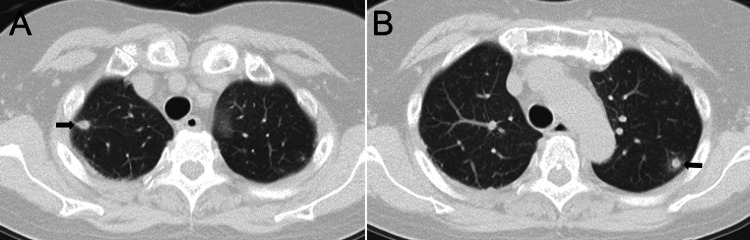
Chest CT A, B: Multiple nodules are observed in the peripheral lungs, with surrounding ground-glass opacities suggestive of a halo sign (arrow). CT, computed tomography
